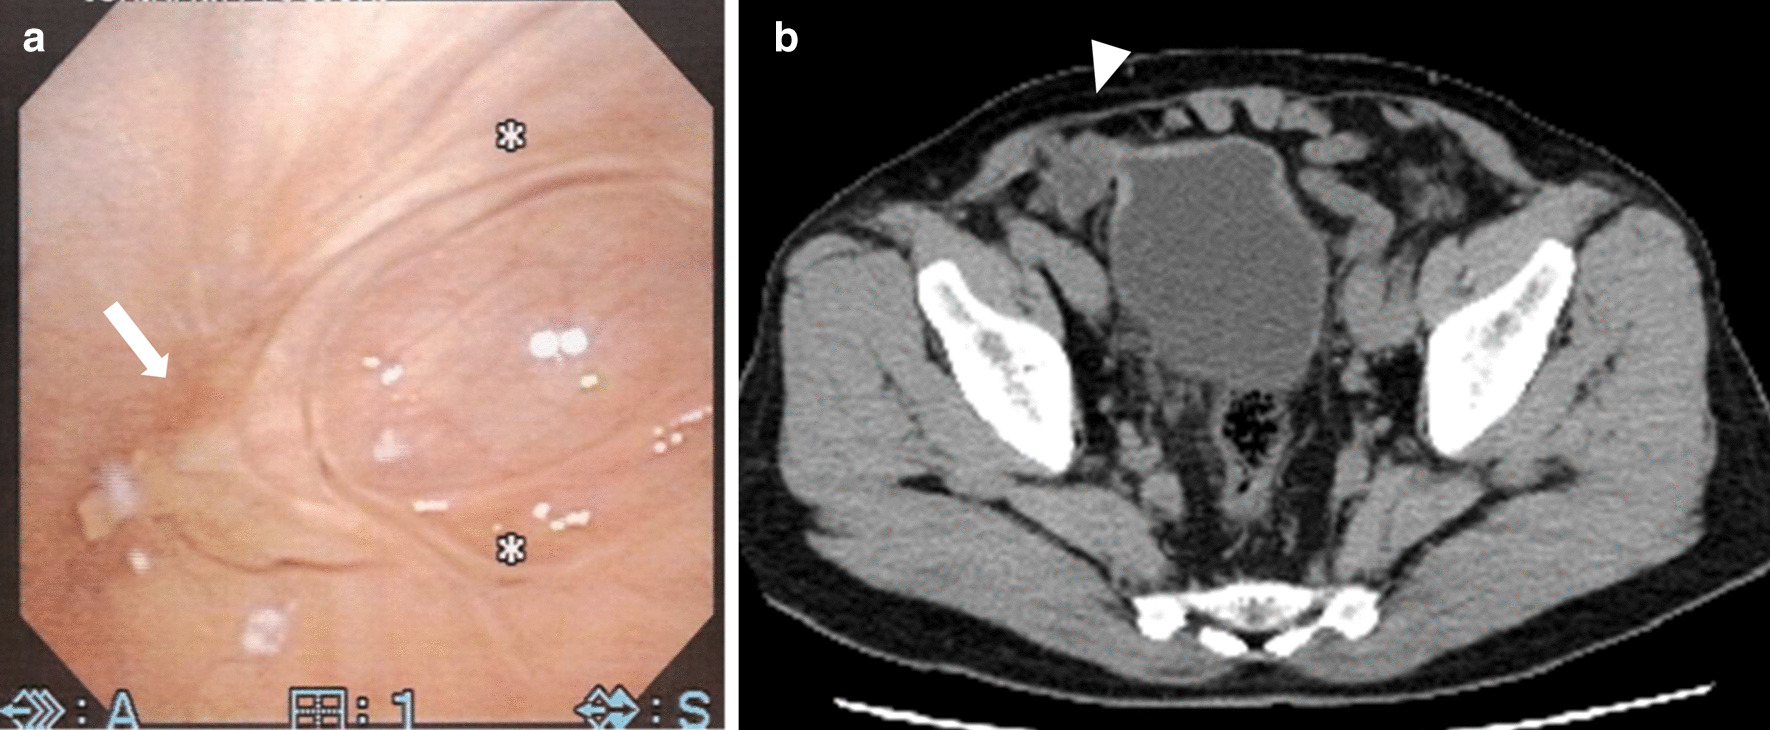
a Cystoscopy 5 months after the second cystoscopy shows a healed fistula (arrow). b Computed tomography scan showing reduced fluid collection (arrowhead)
